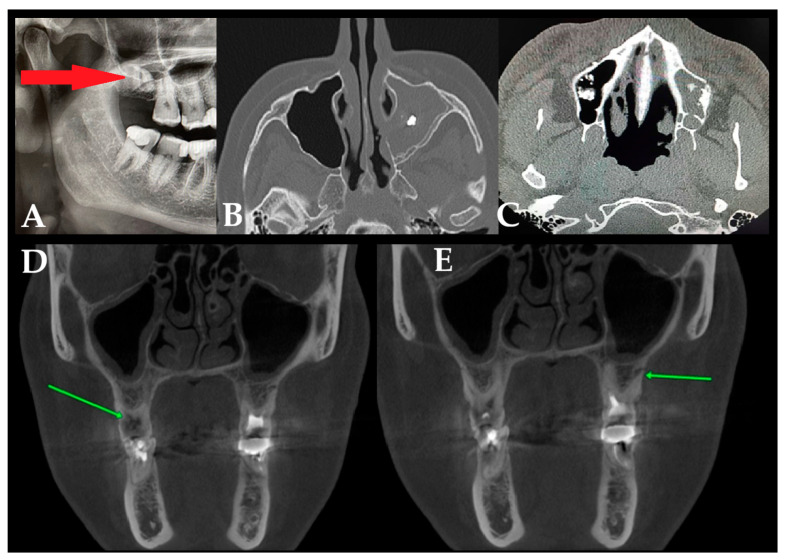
The sources of odontogenic sinusitis (ODS) found in patients’ examination: (A) Retained root associated with maxillary sinusitis, demonstrated on panoramic reconstruction/OPG (red arrow). (B) Endodontic material used for dental root treatment (CBCT axial view). (C) Bone filling material used for socket preservation, bone fillings, sinus lift materials, and other materials that protruded towards the maxillary sinus, like xenograft, allograft or autograft bone materials. In the presented case, the right maxillary sinus was without any changes after the sinus lift procedure. Left maxillary sinus without ventilation with xenograft bone spread within the sinus that caused an ODS. (D,E)—Unfinished and long-forgotten dental treatment: the left arrow indicates a dental crown destroyed by caries, while the right arrow indicates unfinished endodontic treatment (green arrows).
